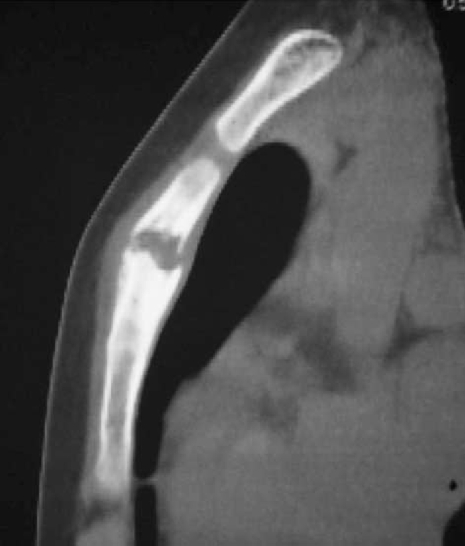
Computed tomography (CT) showed an osteolytic lesion that dissolved both anterior and posterior cortex of the body of the sternum.
